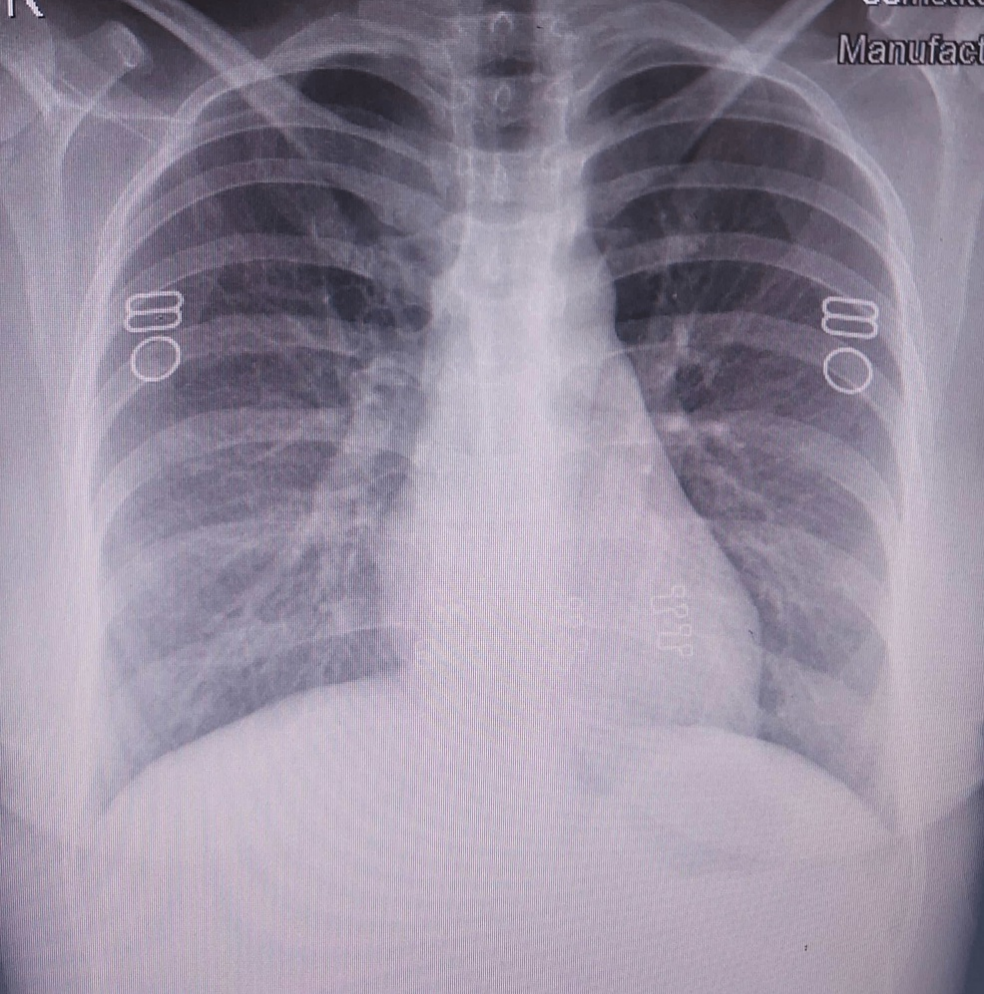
Repeat chest radiograph at the time of discharge showing clear lung fields.
